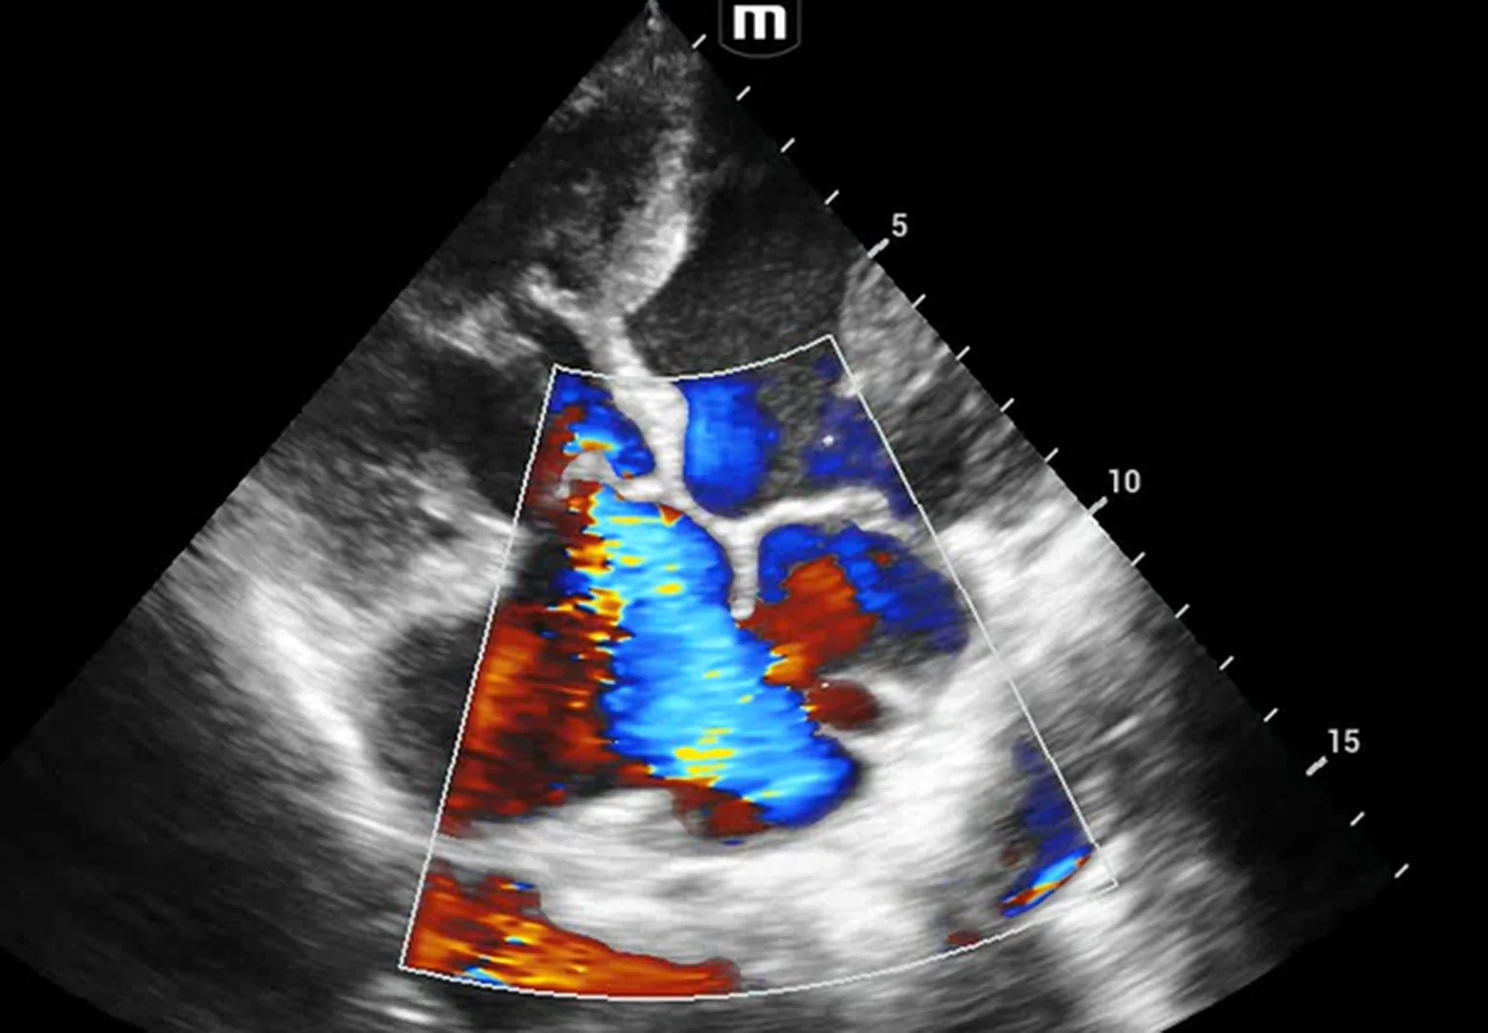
Colour Doppler transthoracic echocardiography demonstrating intracardiac flow and severe tricuspid regurgitation; estimated systolic pulmonary artery pressure was approximately 85 mmHg
